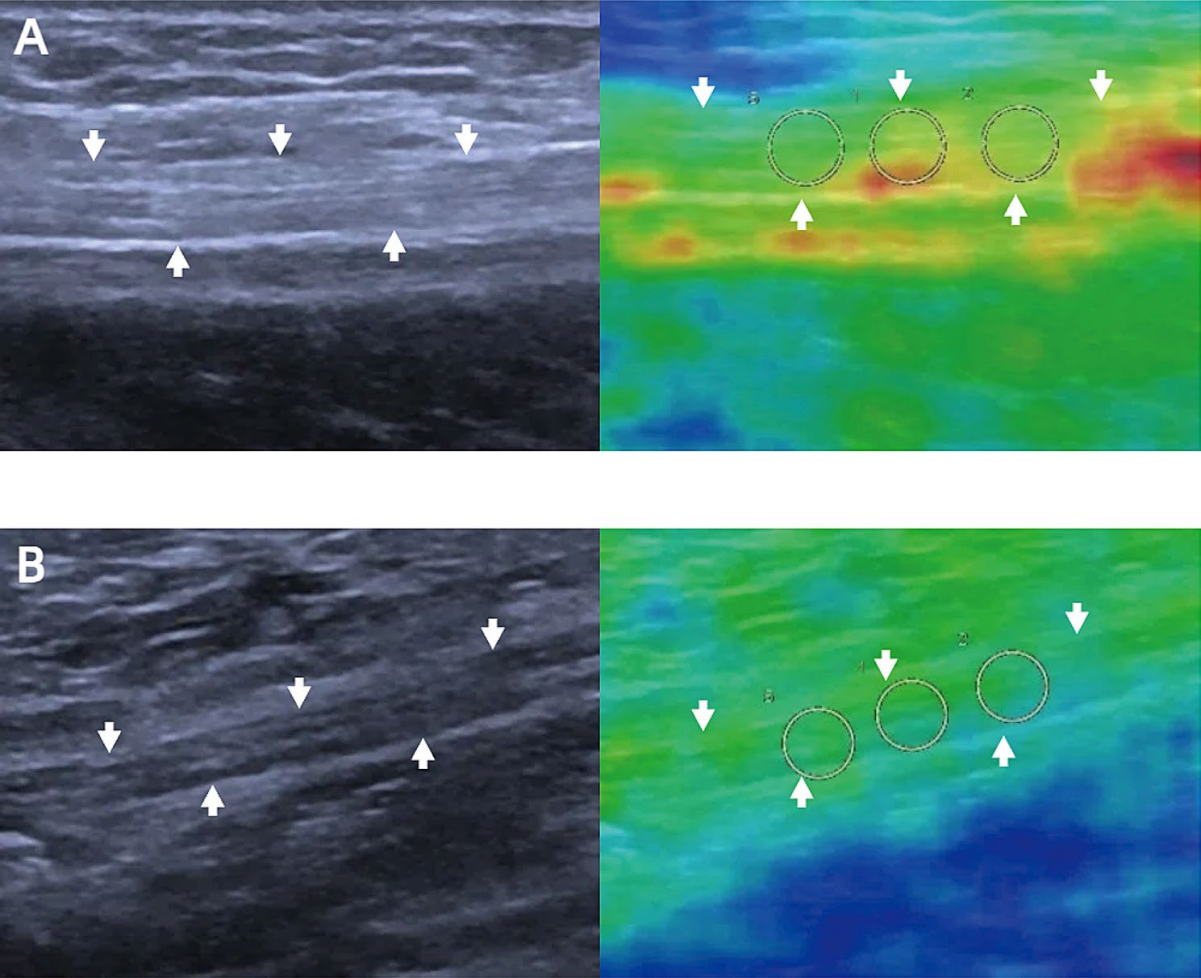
Representative grayscale ultrasound and shear wave elastography images of the tibial nerve (white arrow) (A) A 53-year-old DPN patient with shear wave velocities values of 4.43 m/s, 4.24 m/s and 3.49 m/s. (B) A 63-year-old non-DPN patient with shear wave velocities values of 2.88 m/s, 2.93 m/s and 3.11 m/s. DPN, diabetic peripheral neuropathy; non-DPN, nondiabetic peripheral neuropathy
